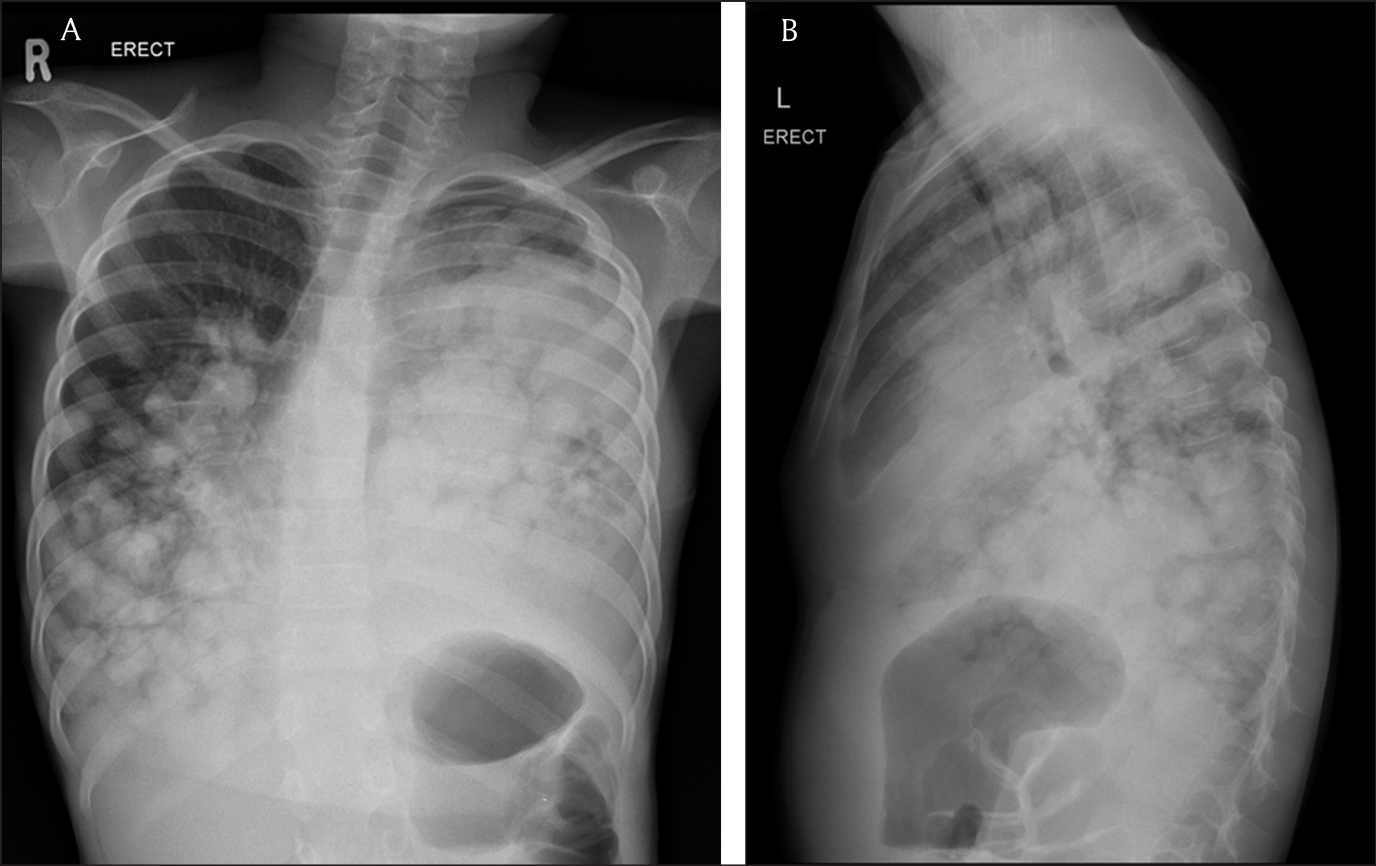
Follow-up frontal and lateral radiographs two weeks later showed interval decrease of the parenchymal hydatid cysts, especially on the left side.
